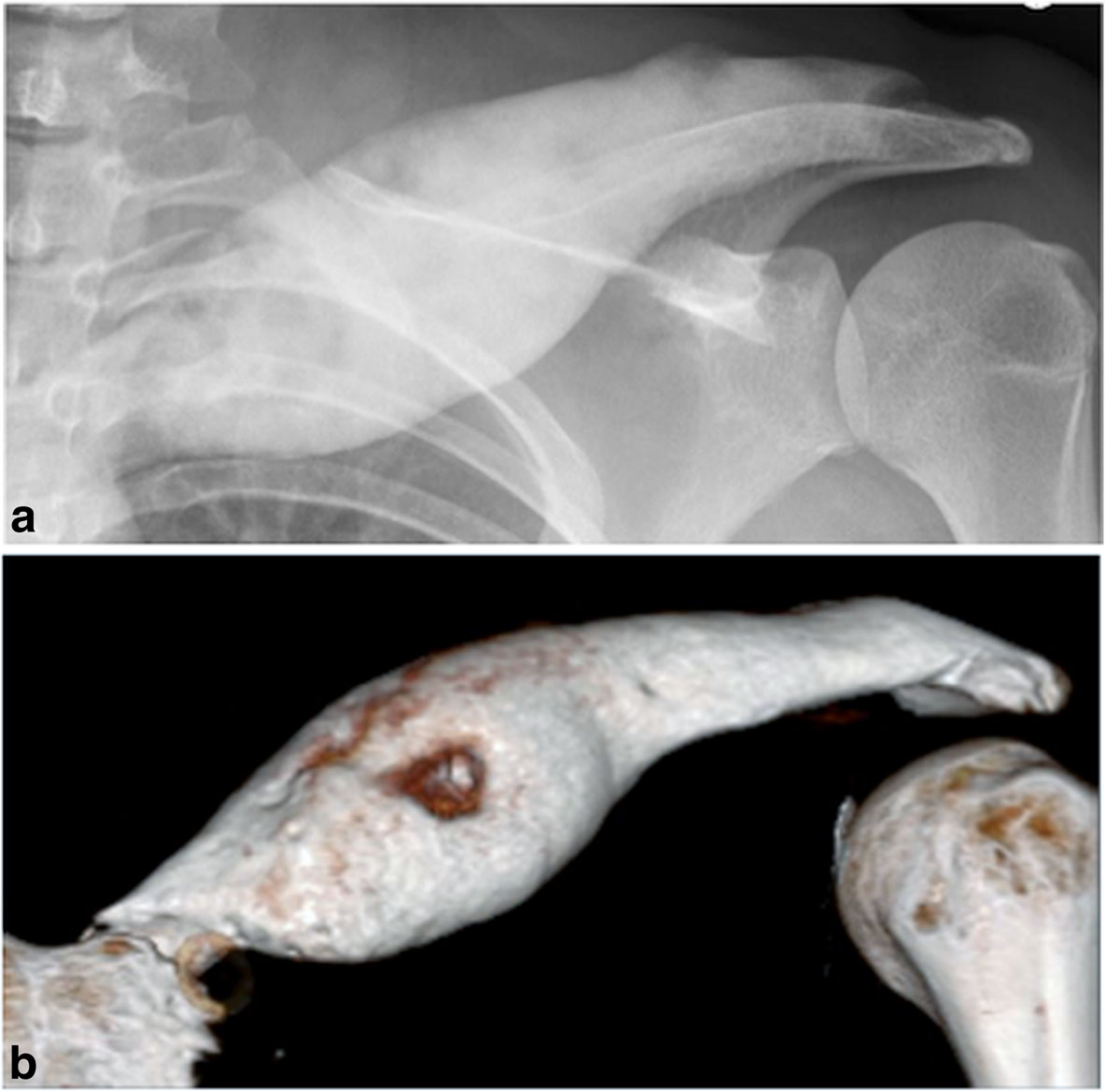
a Anteroposterior radiograph and b volume-rendered computed tomography image of the left clavicle prior to surgical reconstruction
